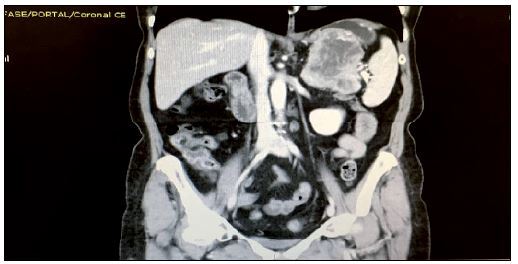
Computed tomography showing a lesion in the tail of the pancreas.
